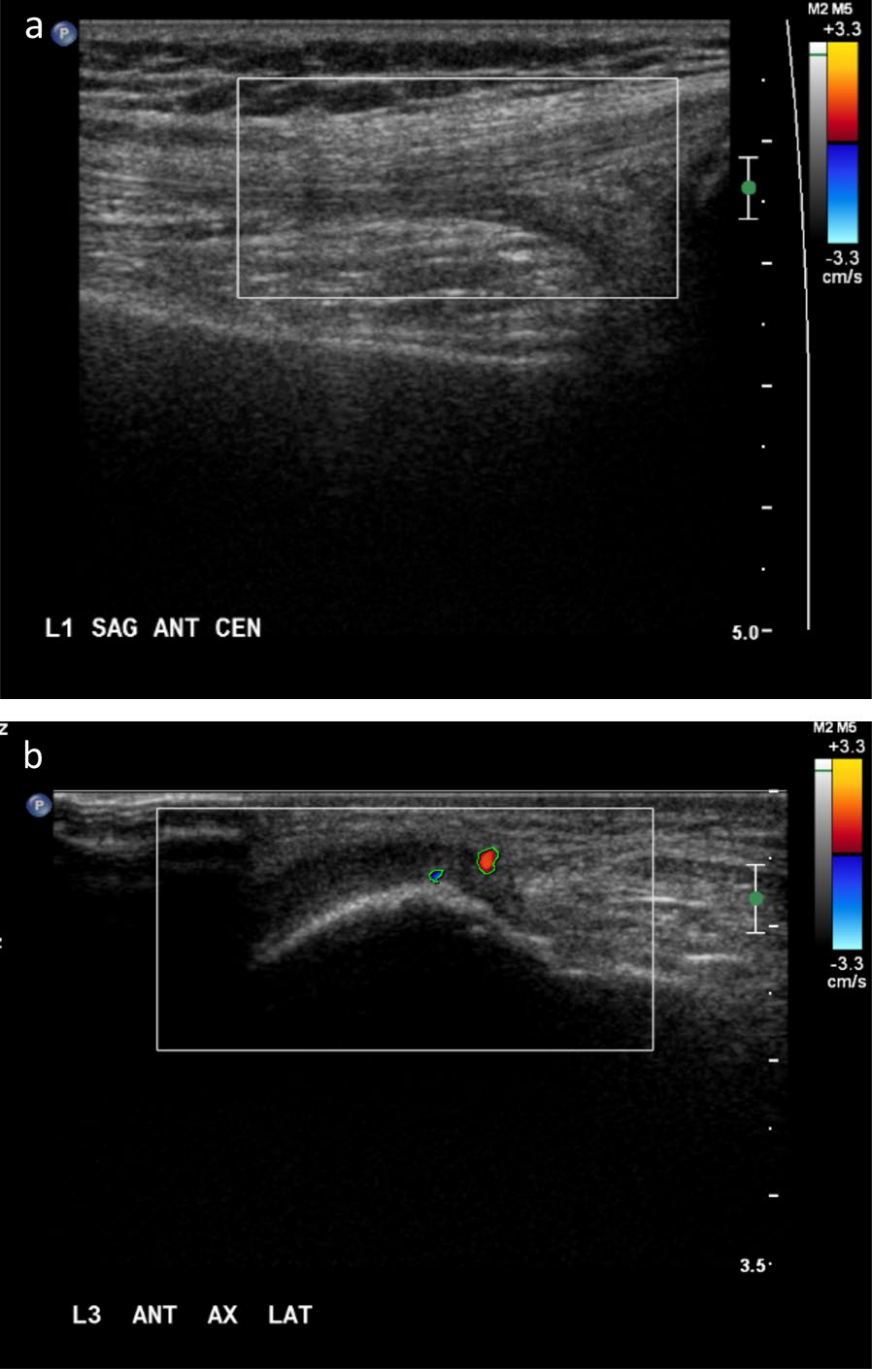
Normal synovial vascularity of joints of patients with hemophilic arthropathy on color Doppler ultrasound. a Thirteen-year old boy with severe hemophilia A and a history of recurrent bleeding into his right knee. Color Doppler sonogram obtained on the sagittal anterior central plane at the L1 level shows no color pixel dot within the color box. Score is 0. Objective area of pixels is 0, score equals 0. b Fourteen-year old boy with severe hemophilia A and a history of recurrent bleeding into his right knee. Color Doppler sonogram obtained on the axial anterior-lateral plane at the L3 level shows 2  color pixel dots (less than 4 dots) within the color box, Score equals 0. The area of color pixels within the color box is 569 units^2^
